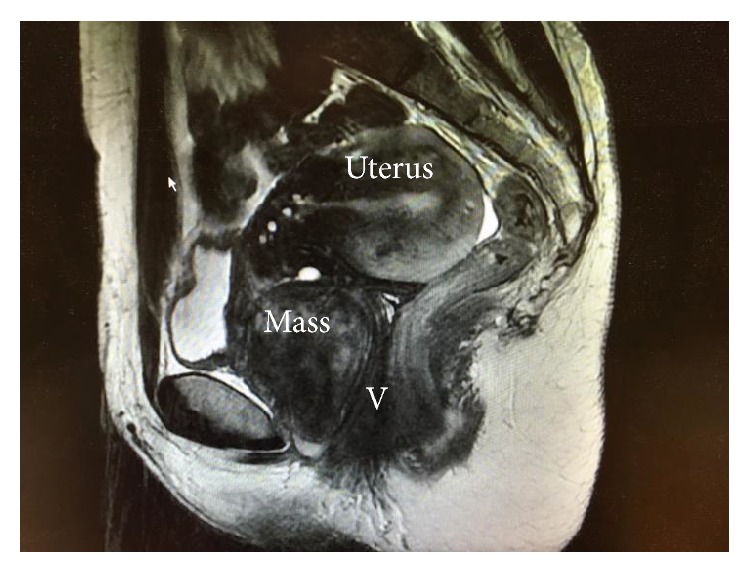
Sagittal section of MRI showing the 45 mm × 50 mm solid mass in the vesicovaginal septum in relation to the uterus, mass, and vagina (V).
